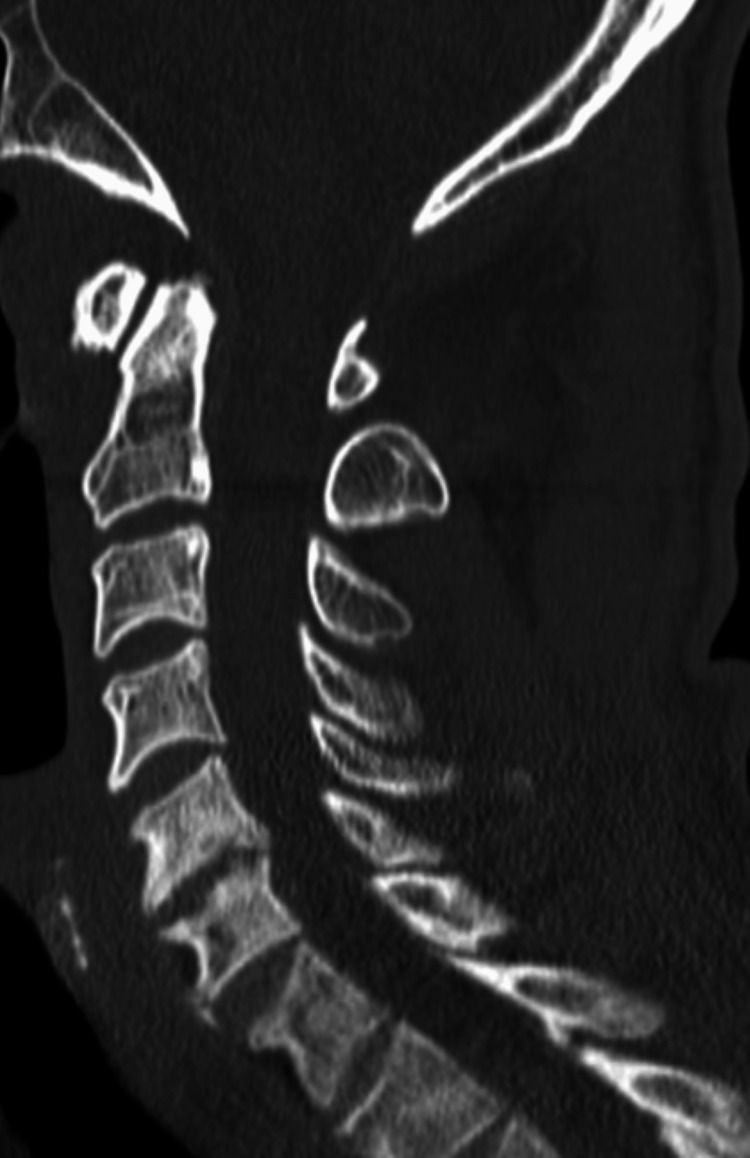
Non-contrast enhanced CT cervical spine showing no acute fractures with only mild degenerative bone changes noted CT: computed tomography
